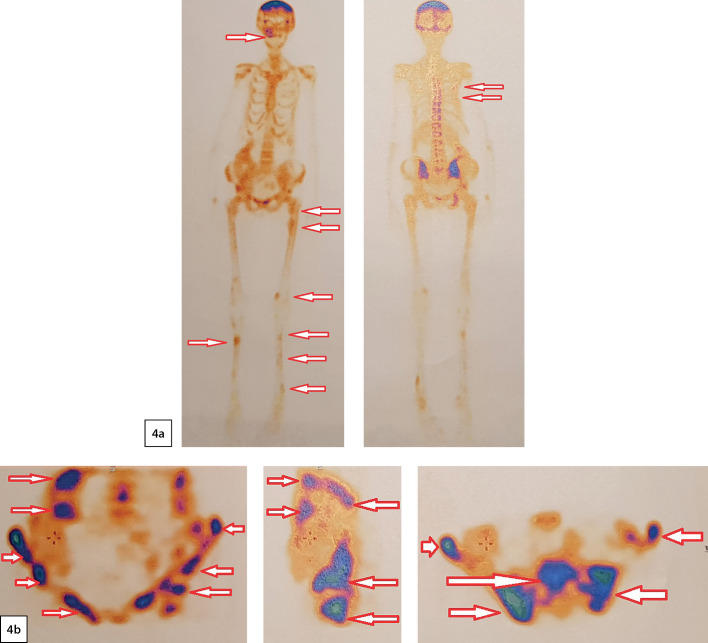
Figure 4. Bone scintigraphy: 4a - scintigraphy of bones in the «whole body» mode: arrows indicate maxilla focal lesion on the right as well as focal lesions in the region of the ribs on the right, lower extremities; 4b - pelvic scintigraphy: arrows indicate multiple lytic lesions in the pelvic bones.
